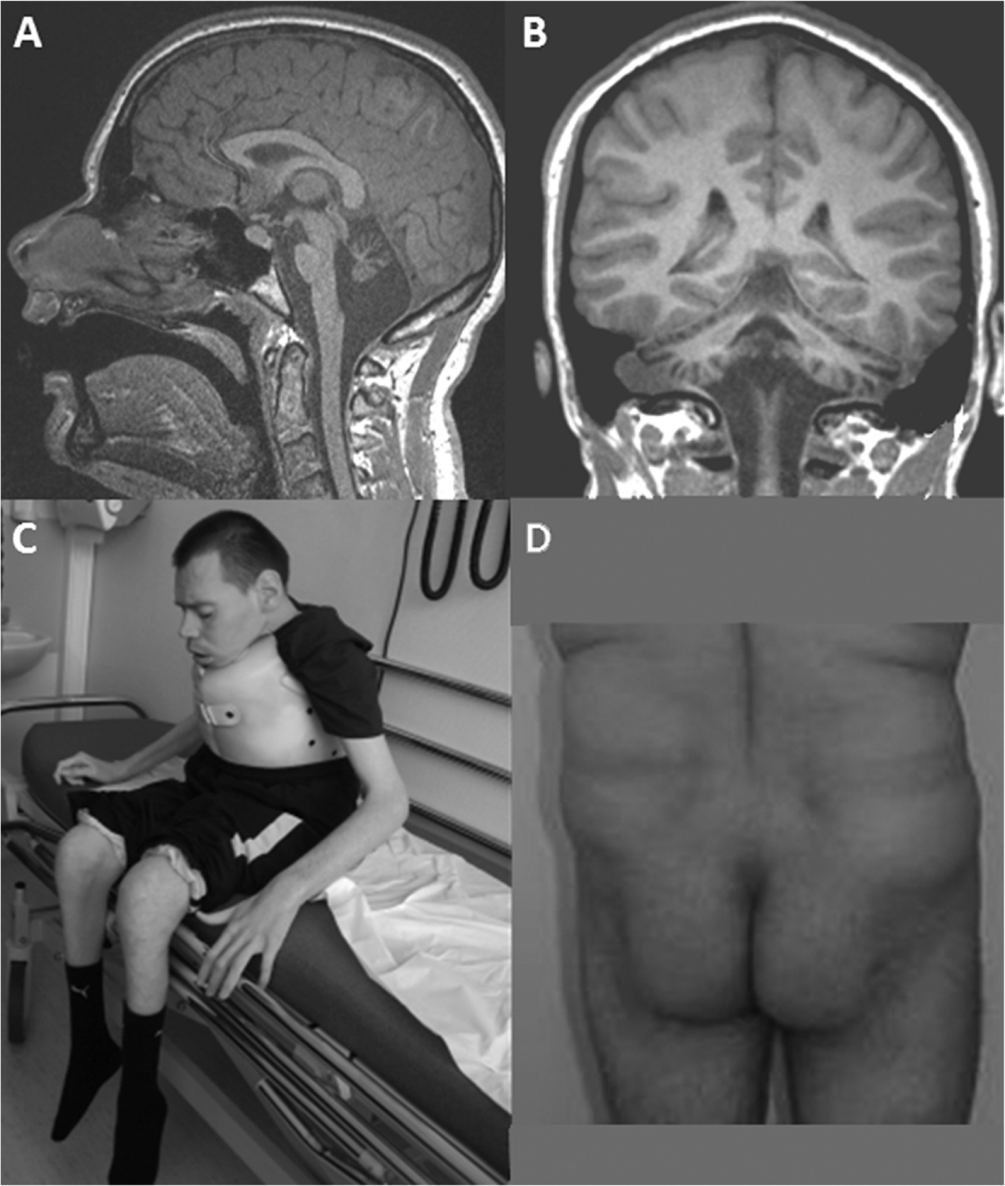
Brain MRI and morphological aspects of adult PMM2-CDG. Upper panel. Brain MRI of patient #19 at 16 years old. T1-weighted sagittal (A) and frontal (B) images showing severe atrophy of the cerebellum, including vermis and hemispheres, and less atrophied pons. Lower panel. Picture of a 32 year-old patient with severe ataxia, neuropathy and severe intellectual disability (C). Picture of a 45 year-old patient showing the abnormal fat repartition typical of PMM2-CDG (D).Epilepsy was diagnosed in 8/23 patients and always started before age 15, mainly between 3 months and 3.5 years of life. Electroencephalographic recordings (EEG) showed diffuse or focal slow background activity in three patients and anterior fast rhythms (without benzodiazepine therapy) in another. Seizures were well-controlled with antiepileptic therapy, mostly with a single drug and with two drugs or more in two-thirds of the cases. Only occasional seizures occurred in adult patients.22/24 patients had delayed development and intellectual disability (ID) of variable severity. 10/15 patients with informative data achieved the ability to read at least a few words and six patients could read correctly and write by hand or with the use of a keyboard. None of these patients had a typical education: four patients followed an adapted program in “normal” schools but the others were enrolled in specialized schools for mentally and physically disabled children. Among 17 patients of working age, one worked as a farm-worker and another obtained an internship in business. Three patients were still working in specialized employment for people with disabilities and four others were waiting for such employment. The eight other patients were dependent on their family at home or attending occupational centers.Signs of peripheral neuropathy were reported for 22 patients and found in 13 after the age 15, mainly abolished osteotendinous reflexes, distal amyotrophy and sensitive alteration. Nerve conduction velocity (NCV) had been measured in nine patients. Two patients had a normal exam, axonal neuropathy was diagnosed in one and demyelinating neuropathy in the six others (exam performed between 1 and 49 years of age). NCV recordings were repeated in three cases, and demonstrated a slow worsening of demyelination over a period of 9 years in one patient and over a period of 3 years in adulthood for the other, with additional involvement of the upper limbs.Psychological aspects. Although PMM2-CDG patients were often described as affable and cheerful, eight patients in our cohort developed psychiatric symptoms, including aggressive behaviour (n = 5), apathy (n = 2) and depression (n = 1). These difficulties could contribute to the loss of autonomy, as exemplified by the depressed patient #22 who lost the ability to walk.
